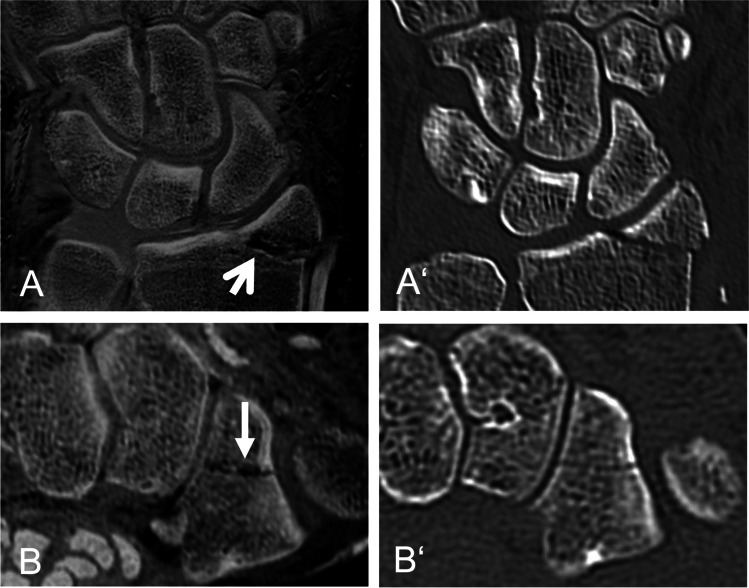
Twenty-three-year-old patient with an acute fracture of the styloid process of the radius (open arrow) and the trapezium (closed arrow). Multiplanar reconstruction in sagittal (A, A‘) and axial plane (B, B‘) based on an inverted 3D T1GRE sequence (A, B) and a CT (A‘, B‘)
